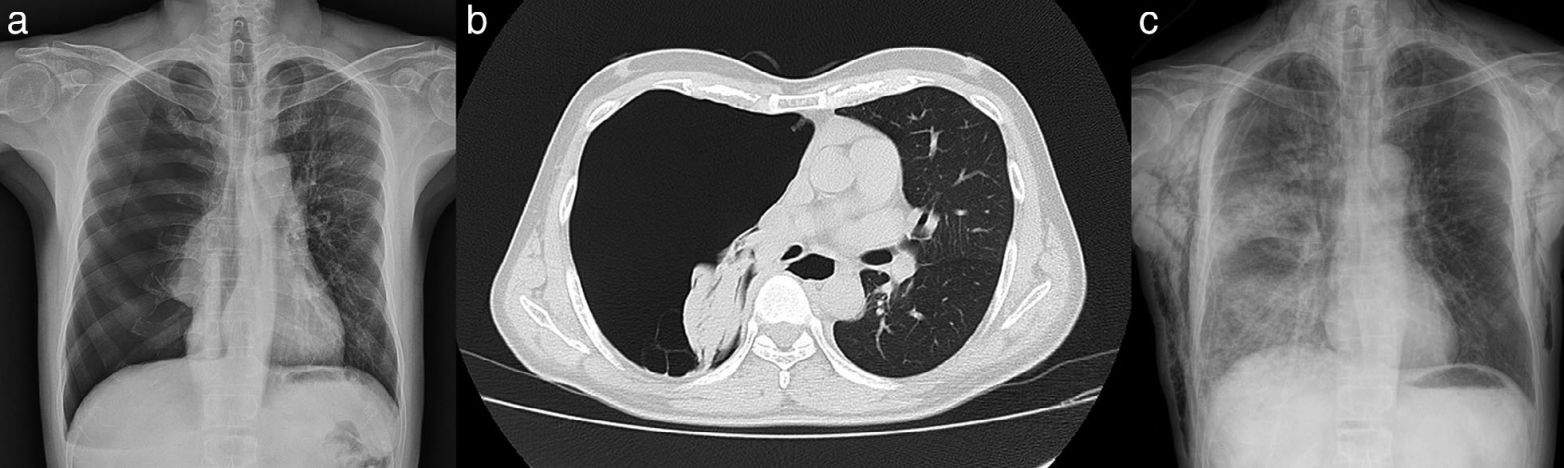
(a) Chest radiograph showed right‐sided pneumothorax, (b) Unenhanced chest CT showed a completely collapsed right lung, (c) Chest radiograph obtained after chest tube insertion showed diffuse consolidation and GGO, suggesting re‐expansion pulmonary edema. Subcutaneous emphysema is visualized. CT, computed tomography; GGO, ground‐glass opacity.
